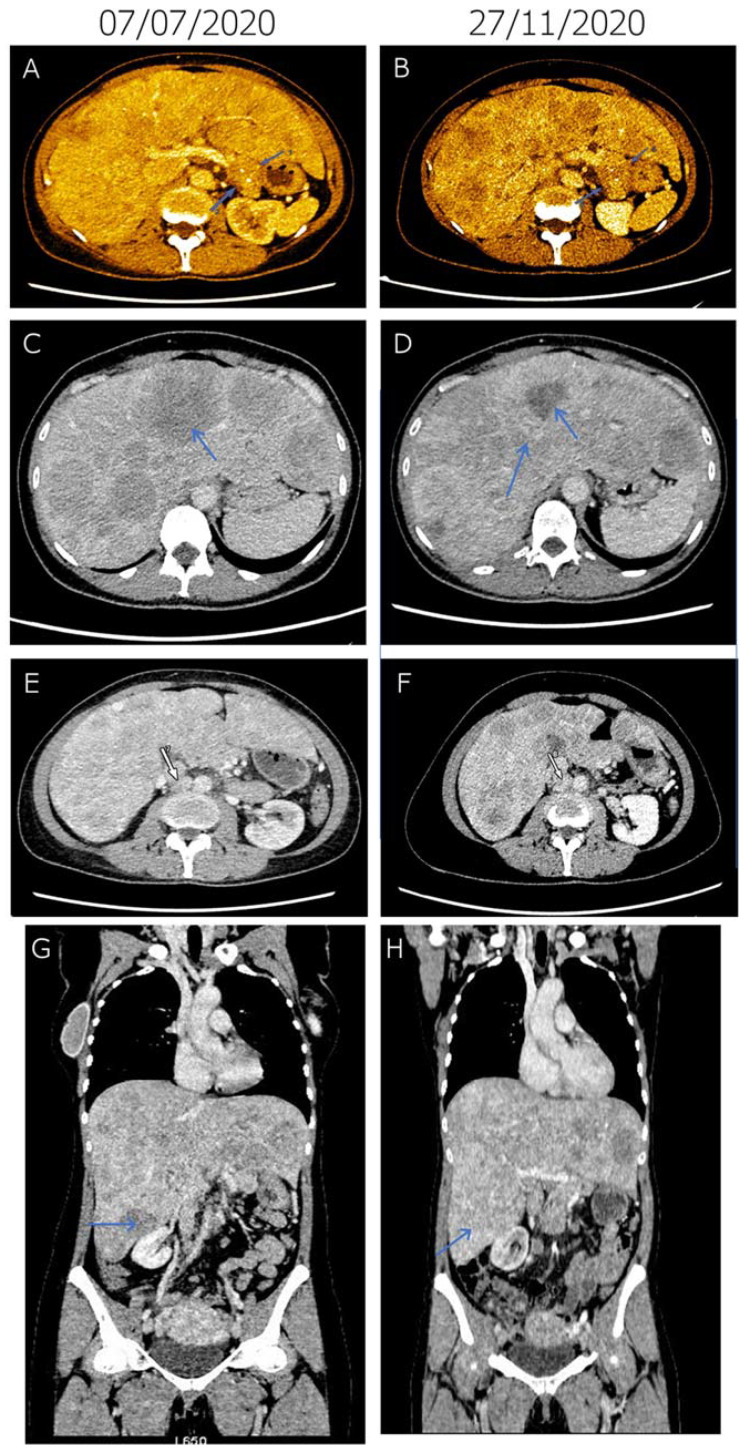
Tumor response achieved by first-line molecularly targeted everolimus therapy in advanced pancreatic neuroendocrine carcinoma. (A–H) Representative contrast CT slices at two time points: 7 July 2020 (at diagnosis, left) and 27 Novermber 2020 (right). At diagnosis (7 July 2020), a 22 × 27 mm, inhomogeneous, oval soft-tissue mass could be observed behind the pancreas tail (arrows), which could not be distinguished from the outline of the pancreas with certainty (A). Two tiny spots of calcification were also present. On 27 Novermber, the mass was detected with unchanged status and of the same size (arrows), indicating stable disease (B). At diagnosis (7 July 2020), the liver was significantly enlarged. Major parts of the liver area were filled with occasionally conflating hypodense regions of blurred outlines (arrow), indicating multiple metastases (C). Morphology of the liver metastases was altered by 27 Novermber due to changes in contrast agent dynamics (arrow), which is a consequence of the therapy without meaningful changes in size or number (D). At diagnosis (7 July 2020), in the retroperitoneal space, lymph nodes (arrow) increased in number and size were detected, indicating lymph node metastases (E). At the lower part of the right kidney, the short diameter of the largest node was 20 mm. On 27 Novermber, lymph nodes (arrow) were in regression, the diameter of the largest node decreased to 17 mm (F). (G,H) Compared to the 7 July 2020 baseline (G), metastases in the lymph node and the liver (arrow) regressed and morphologically altered, respectively (H).
